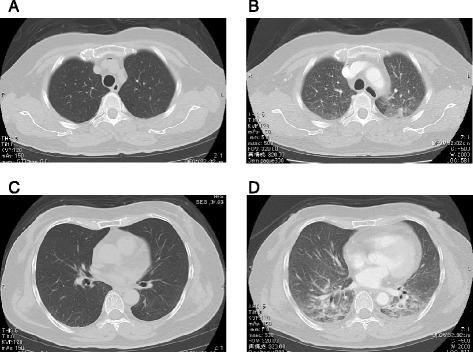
Computed tomography scans. (A, C) The plain computed tomography scan on admission. (B, D) The contrast computed tomography scan at hemodynamic deterioration. A and B were the same level of the body. C and D were the same level of the body.
